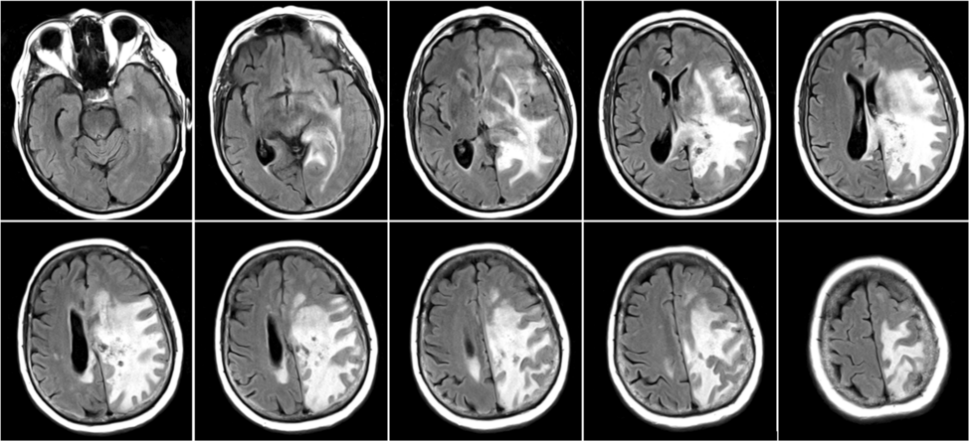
Fluid-attenuated-inversion recovery (FLAIR) sequence of the initial MRI
